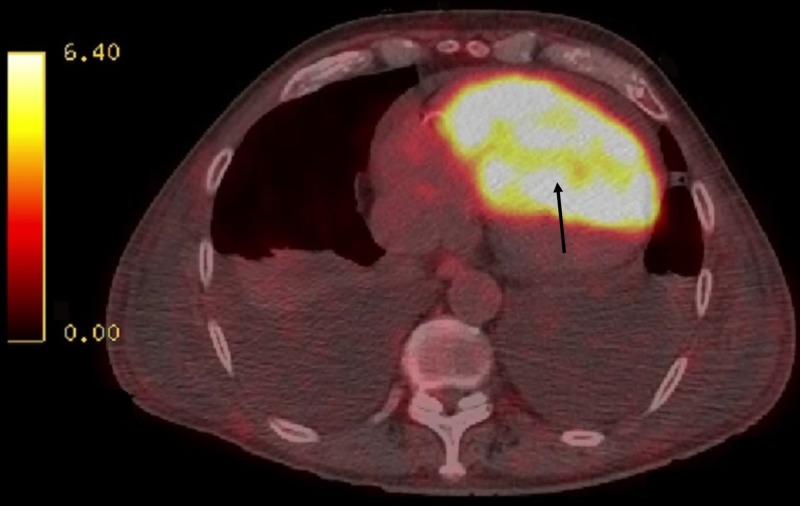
Positron emission tomographic/computed tomographic scan shows increased fluorodeoxyglucose activity within the right ventricular mass.
